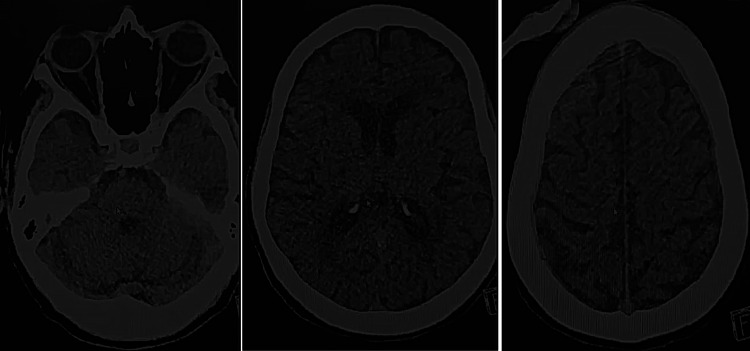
Non-contrast CT Head (Axial Slices) Three axial non-contrast CT images demonstrating no acute intracranial pathology.
